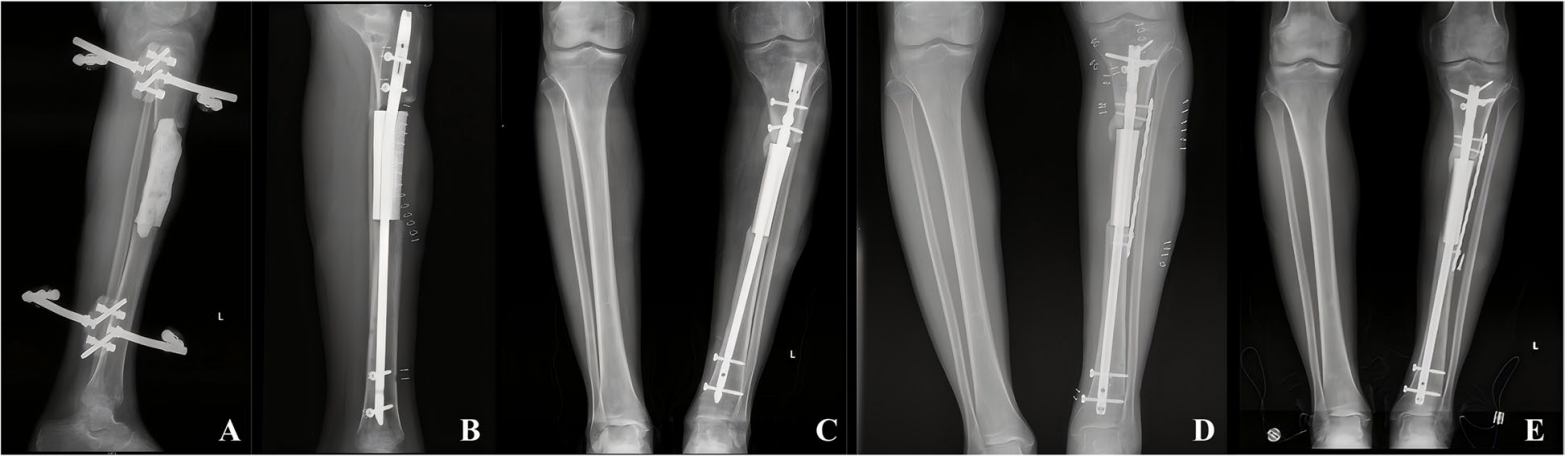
Case 4. A 37-year-old patient who suffered prosthesis displacement and limb deformity due to an intramedullary nail locking screw fracture. A, B Lateral radiographs of the first treatment. C The patient complained of pain at 6-month follow-up, angular displacement of the prosthesis and locking screw fracture can be observed on X-ray. D Revision surgery was performed with intramedullary nail replacement and internal plate fixation. E Showing the stability of the reconstruction at 3-year follow-up after revision surgery
